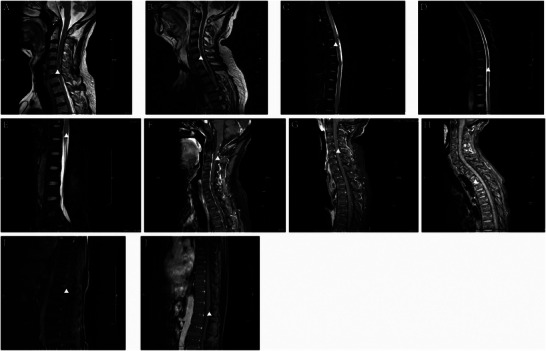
Different manifestations of the cervical, thoracic, and lumbar spinal cord on MRI. (A–B) The cervical spinal cord shows cord‐like and patchy high signal intensity on the T2 sequence; (C–D) The thoracic spinal cord shows linear, patchy, and patchy high signal intensity on the T2 sequence; (E) The lumbar spinal cord shows a slightly thickened spinal cord membrane on the T2 sequence; (F) The cervical spinal cord shows significant enhancement; and (G–I) The cervical, thoracic and lumbar spinal cords show different degrees of enhancement.
